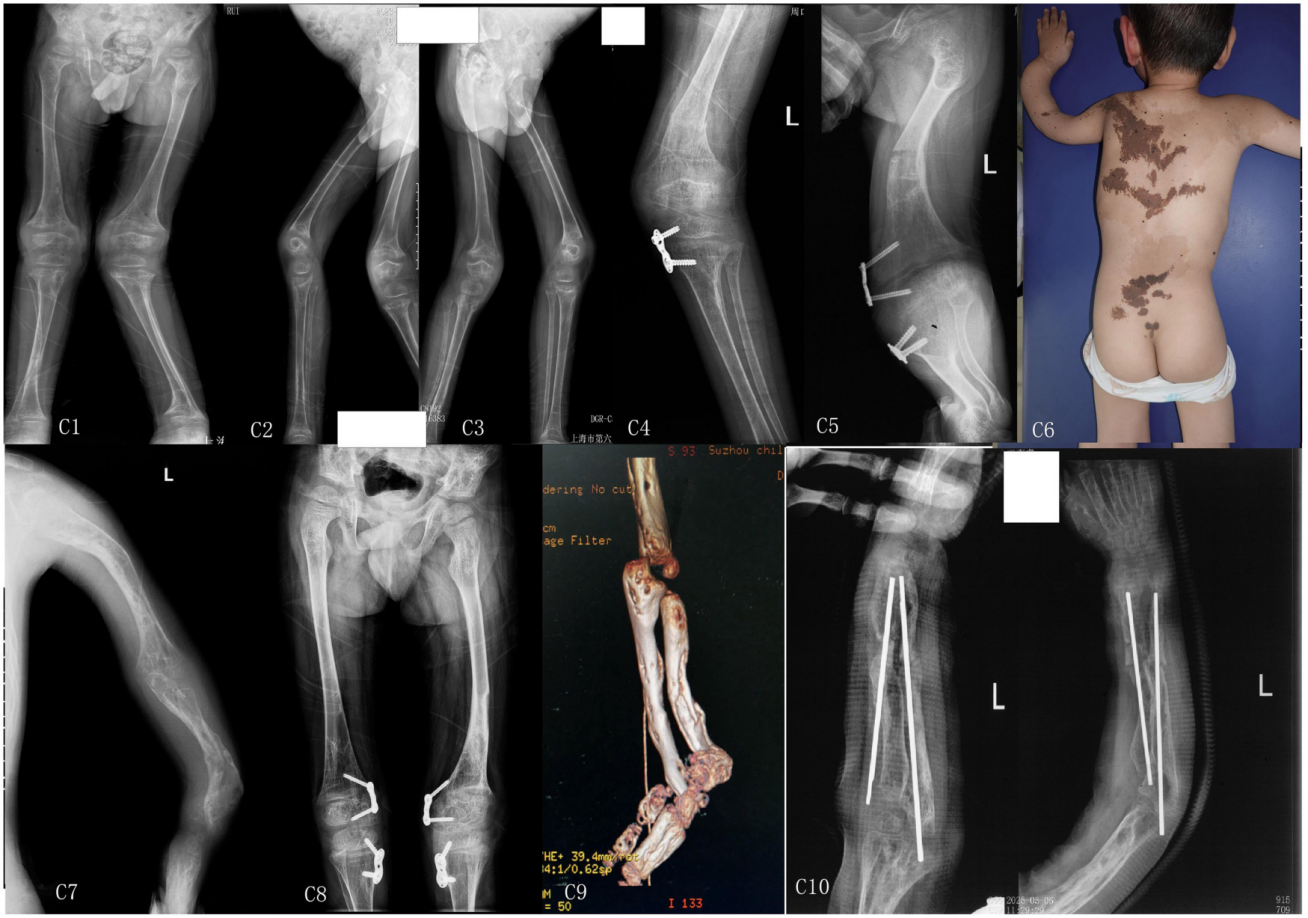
Radiographic and clinical progression of Case 3. C1–C3. A 5-year-old male patient presented with anteroposterior and lateral radiographs of the bilateral lower limbs, which revealed marked valgus deformity of the left knee, osteoporosis in both lower limbs, and abnormal fractures with deformities. C4. The patient had a left distal femoral fracture. The medial growth plate of the left tibia exhibited signs of growth arrest. C5. At age 5, the patient underwent medial femoral and tibial epiphyseal arrest at an external hospital, with a midshaft fracture of the left femur. C6. At age 7, the patient exhibited obvious bowing deformity of the left forearm and multiple melanocytic nevi on the back and buttocks. C7–C9. x-rays of the left humerus, ulna, and radius showed bone abnormalities, with distal left radius bowing deformity, as well as bilateral femoral bone abnormalities with deformity. CT scans revealed ulnar and radial bone abnormalities, with distal radius bowing accompanied by cavitation. C10. Two months following multiple osteotomies and intramedullary nailing of both the radius and ulna, follow-up x-rays demonstrated correction of the radial and ulnar deformities, satisfactory forearm alignment, and successful healing at the osteotomy sites.
